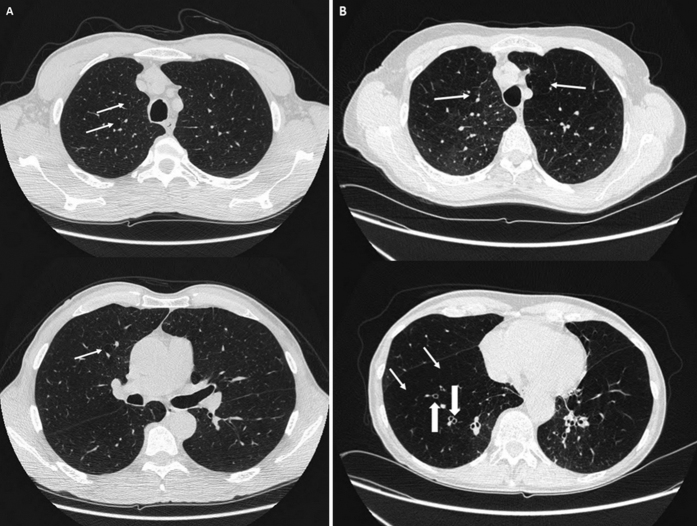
High resolution computed tomographic scans of patients III:3 (A) and III:2 (B). On CT scans, note bronchiectasis, diffuse bronchial wall thickening (large arrows), air trapping and emphysema bullae (thin arrows). These lesions are localized at the basal part of the lung, and not at the apex as noted in smokers. Patient III:2 uses domiciliary oxygen.
